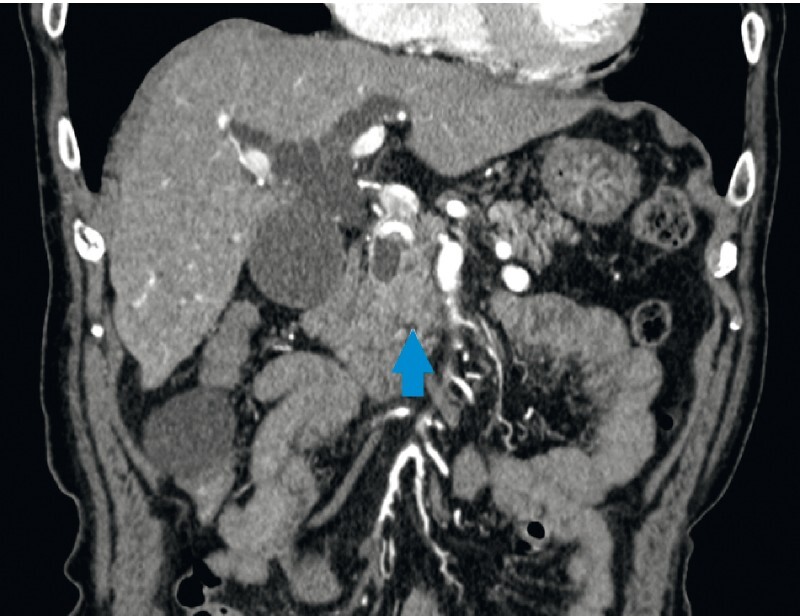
Fluoroscopic images from endoscopic ultrasound-guided hepaticogastrostomy (EUS-HGS) performed for biliary obstruction in an 80-year-old man with pancreatic head cancer.
